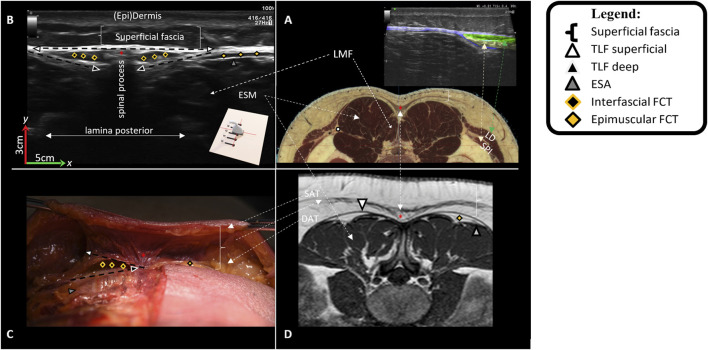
Lumbodorsal fascia anatomical tissues from a transversal view. In this figure, Image (A) represents a transverse MRI image from a Visible Human Male, showing the lumbar tissues at the height of L2-3. Image (B) represents a transverse ultrasonographic image of a healthy individual at the height of L2-3. Image (C) represents a transverse image of a freshly frozen human male cadaver dissected at the height of L2-3. Image (D) is an MRI image (T1, TR 707, TE 11) at the height of L2-3 of a nonspecific low back pain female patient. Image (A) displays the following lumbar tissues: (epi)dermis, superficial fascia, SAT (superior adiposity tissue), DAT (deep adiposity tissue), LD (latissimus dorsi muscle), SPI (serratus posterior inferior muscle), LMF (lumbar multifidus), ESM (erector spinae muscle), *CIFT (central interfascial triangle), and lateral raphe within the TLF. Certain tissues, while not clearly visible in Image (A), become visible in Images (B–D). These include the TLF (thoracolumbar fascia, consisting of superficial and deep lamina), interfascial fat lying between the superficial and deep lamina of the TLF, and epimuscular fat lying between the TLF and the erector spinae aponeurosis (ESA).
